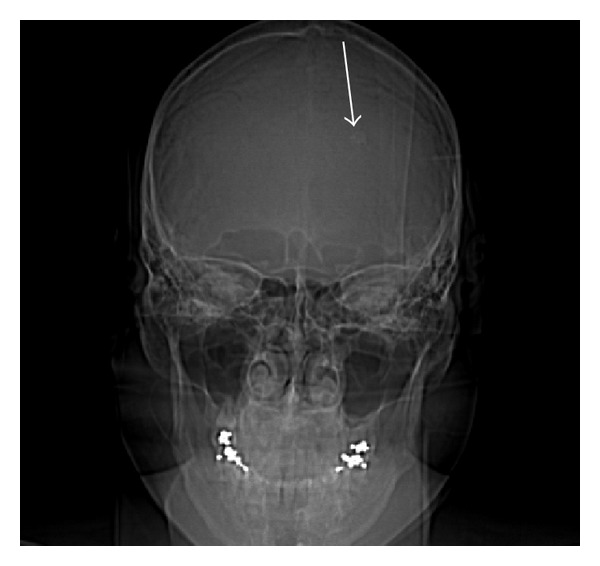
Anteroposterior radiograph of the skull demonstrating a focus of calcification to the left of the midline (arrow).
